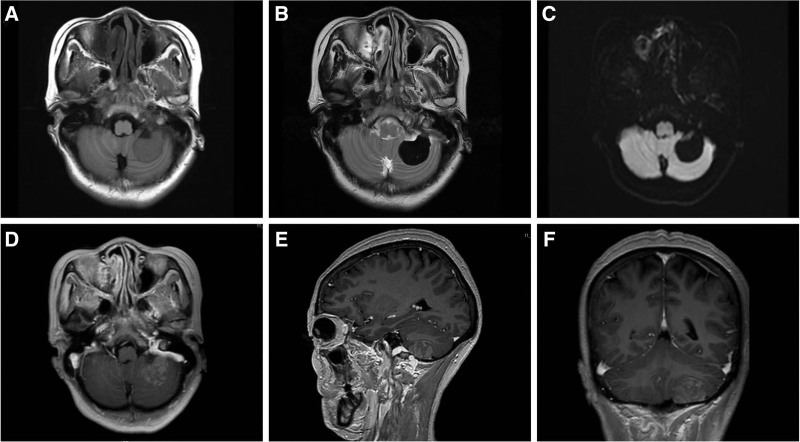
The brain MRI demonstrated a 3.0 cm × 2.1 cm × 2.1 cm ovoid mass in the left occipital. The tumor showed intermediate-slightly decreased signal intensity in T1WI (A) and significantly decreased signal intensity in T2WI (B). Axial (C) DWI did not show diffusion restricted areas. Axial (D), sagittal (E), and coronal (F) enhanced T1WI showed heterogeneous and strong enhancing tumor, connecting with the adjacent meninges by a wide base and compressing the left cerebellar hemisphere. DWI = diffusion weighted imaging, MRI = magnetic resonance imaging, T1WI = T1 weighted images, T2WI = T2 weighted images.
